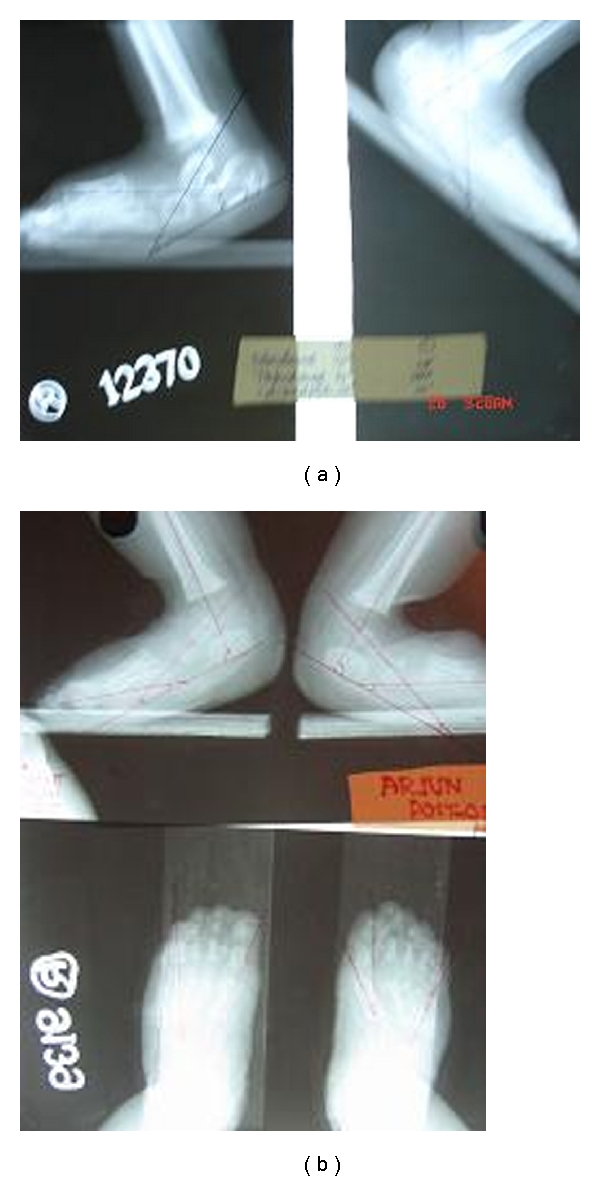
(a) X-rays AP and Lateral (stress dorsiflexion) of feet showing extend of various abnormal radiographic angles preoperatively. (b) X-rays AP and Lateral (stress dorsiflexion) of feet showing extend of various abnormal radiographic angles postoperatively.
